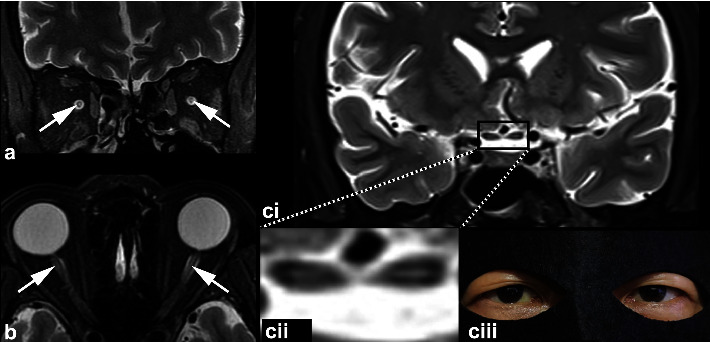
MRI identified characteristic central hyperintense T2 signals in patients with methanol-induced optic neuropathy. (a) Coronal T2 image of patient 3, demonstrating hyperintense signals (arrows) in both orbital optic nerves as “target sign.” (b) Axial T2 image of patient 6, demonstrating hyperintense signals (arrows) in both orbital optic nerves as “neutral axis sign.” (c) (i, ii) Coronal T2 image of patient 4, demonstrating hyperintense signals in both cranial optic nerves as “mask sign” (iii).
